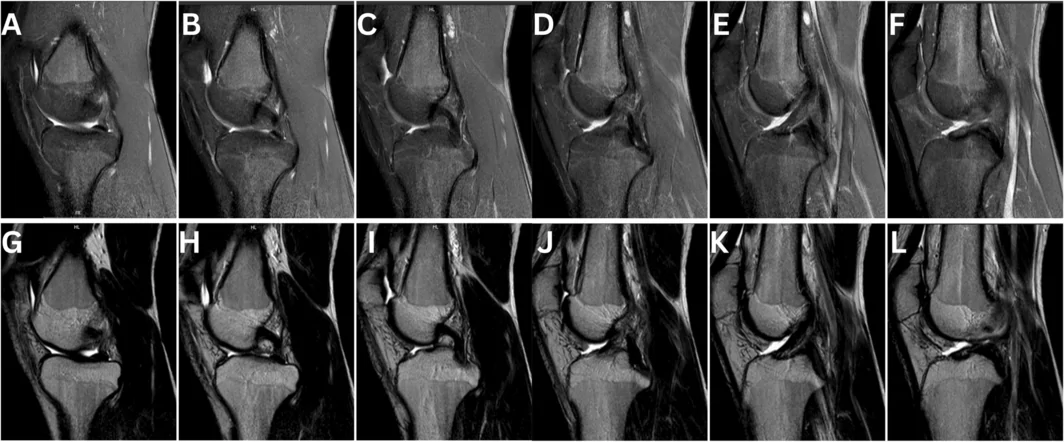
Follow‐up sagittal MRI at 12 months. Fat‐suppressed proton density–weighted (A–F) and non‐fat‐suppressed T2‐weighted (G–L) images show restoration of a continuous, taut, low‐signal ACL band with normal oblique orientation and intact femoral and tibial attachments. The prior discontinuity, fluid‐filled notch, and wavy ligament remnant are no longer evident. Only trace joint fluid is present; the PCL and menisci are unremarkable.
